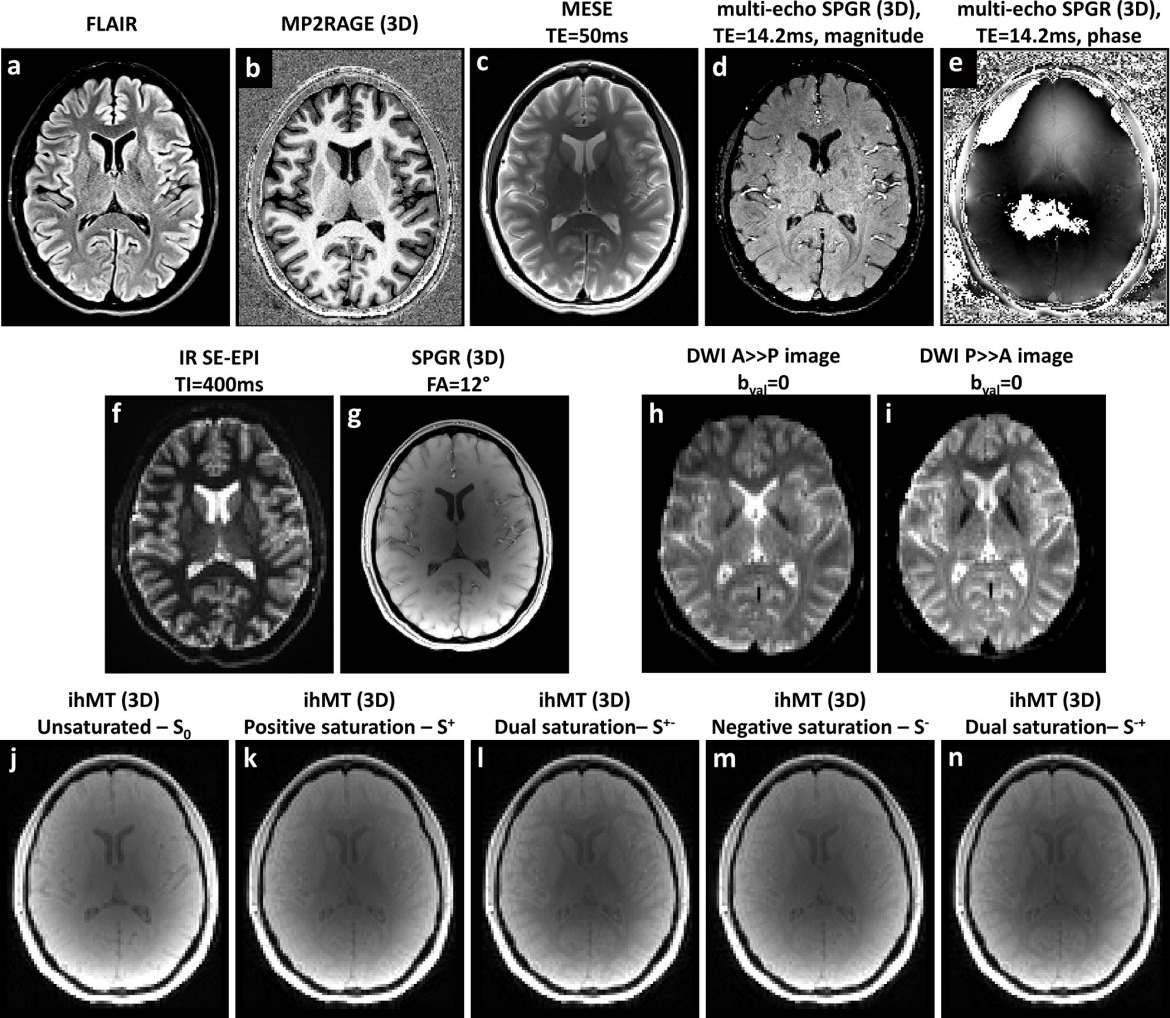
Sample contrast-weighted images for a single volunteer (F, 31 y/o). (a, b) Anatomical scans using FLAIR and MP2RAGE scans. Relaxation weighted images include (c) T_2_-weighted multi-echo SE (MESE); (d, e) T_2_* weighted multi-echo Spoiled-GRE (TE = 14.2 ms) magnitude and phase images; (f) T_1_-weighted inversion recovery SE-EPI; and (g) T_1_-weighted single-echo spoiled-GRE (flip angle = 12°). (h, i) Diffusion-weighted images acquired using two phase-encoding directions: anterior-to-posterior and posterior-to-anterior. Inhomogeneous MT (ihMT) images: (j) Unsaturated M_0_, (k) single positive saturation S^+^, (l) Dual saturation S^+-^, (m) single negative saturation S^-^, and (n) Dual saturation S^-+^.
